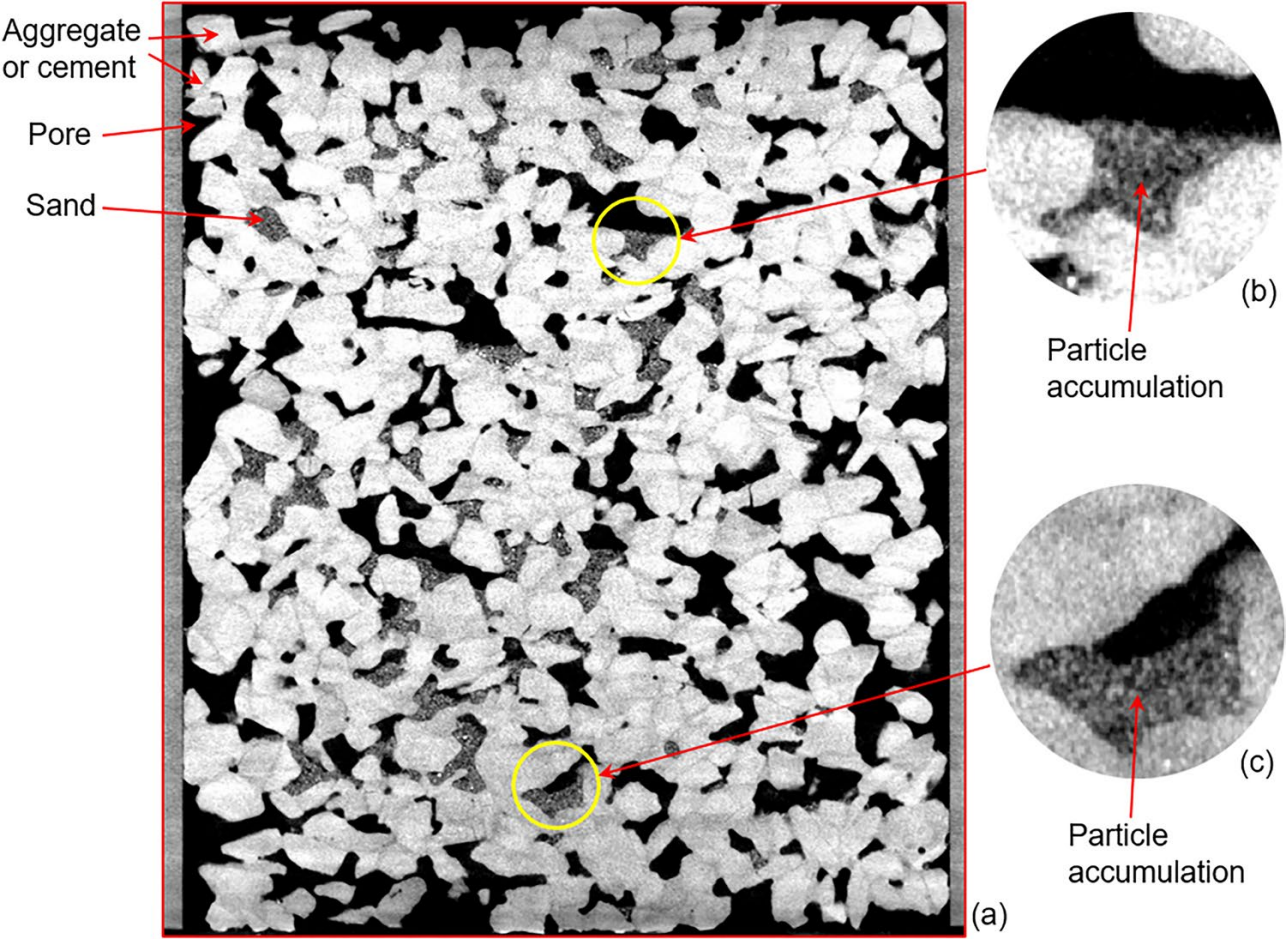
The CT image of the medium sand-clogged sample after all 30 washing cycles: (a) vertical image; (b) particle accumulation-clogging; and (c) particle accumulation-clogging.
